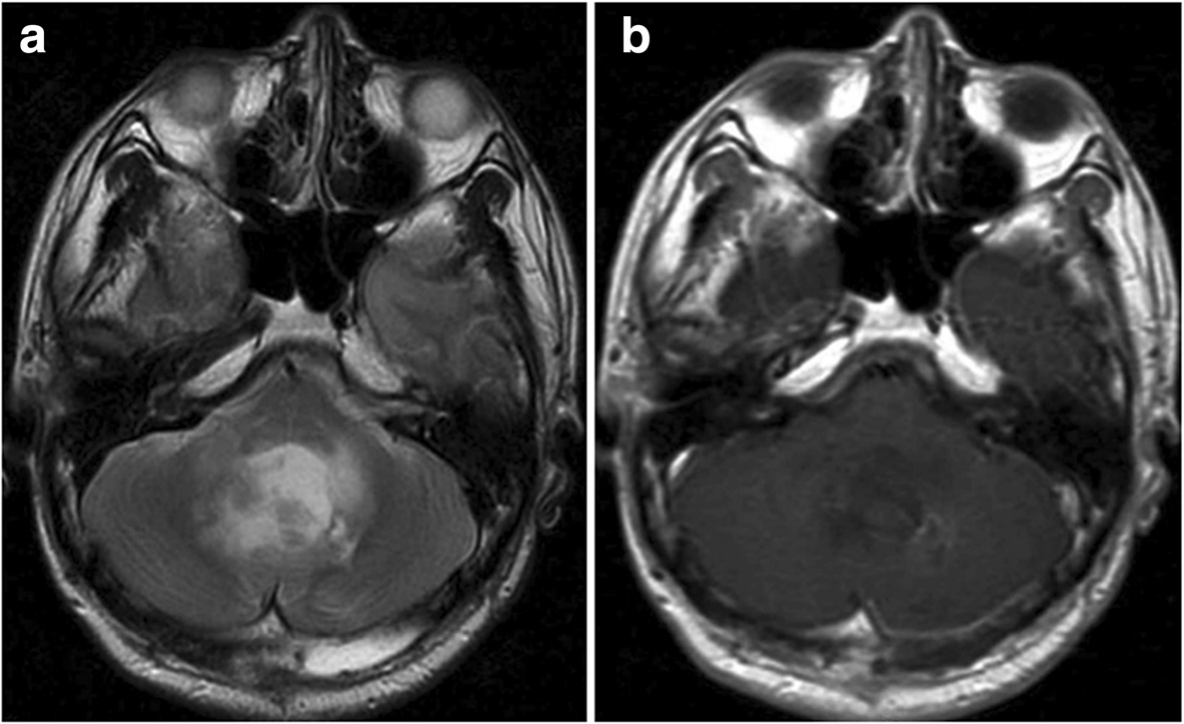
MR images at 1 week after the second surgery revealing total resection of the cerebellar tumor. Axial T2-weighted (a) and Gd-enhanced T1-weighted (b) MR images. Brain edema around the vermis still moderately persists, and the fourth ventricle remains obstructed
